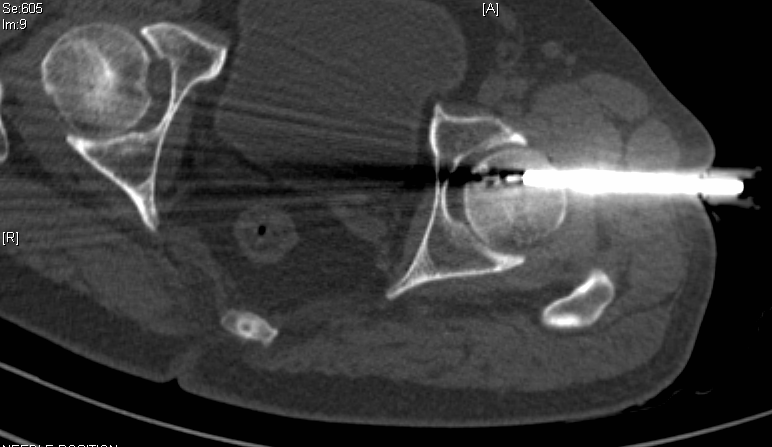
Axial computed tomography section with radiofrequency ablation (RFA) needle placed within the drilled tract.
